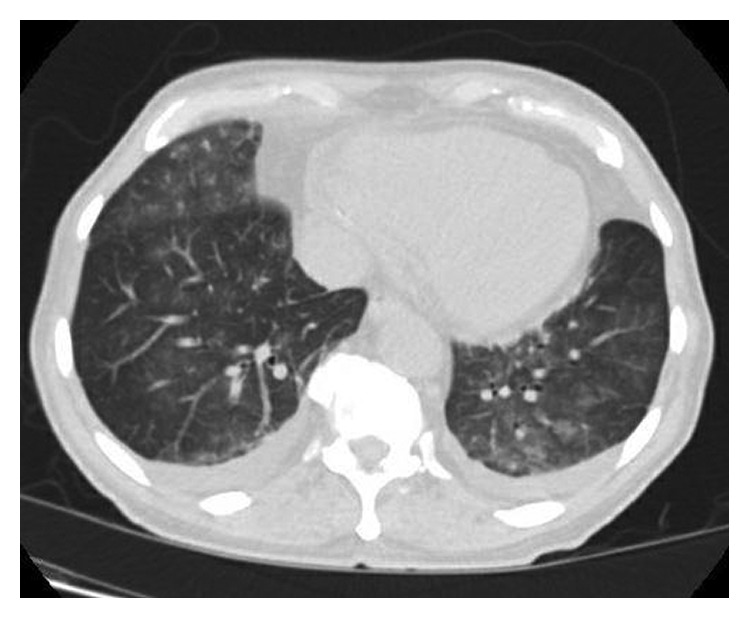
High-resolution CT showing bilateral ground glass opacities and bilateral pleural effusion.
